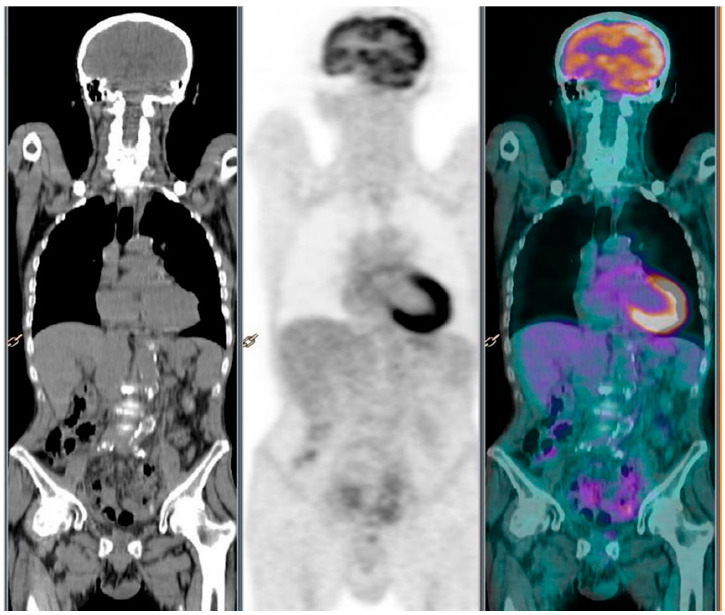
The PET/CT scans performed in December 2017 do not show pathologic masses with high glucose metabolism.
